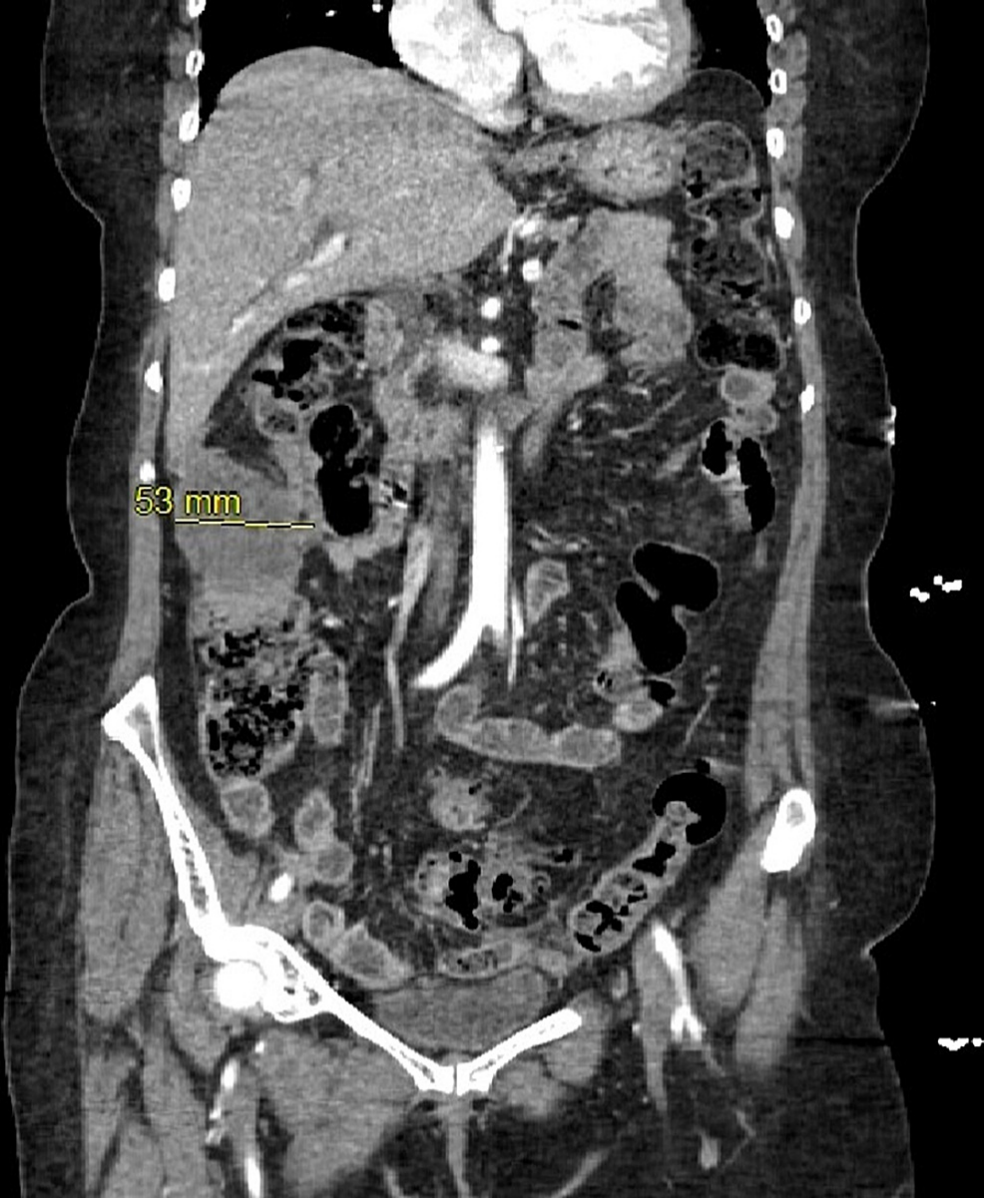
Computed tomography (9CT) abdomen Yellow arrow indication 5.3 cm mass
